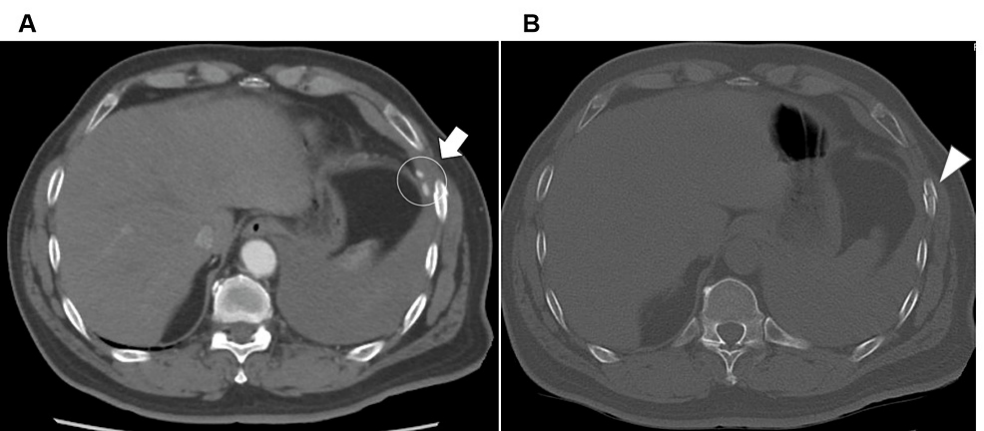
Contrast-enhanced CT scan of the chest performed at the referring hospital. A: Axial scan of arterial phase showing a left pleural effusion and extravasation near the intercostal space of the seventh rib fracture. B: Axial scan of the bone window showing the seventh rib fracture.
